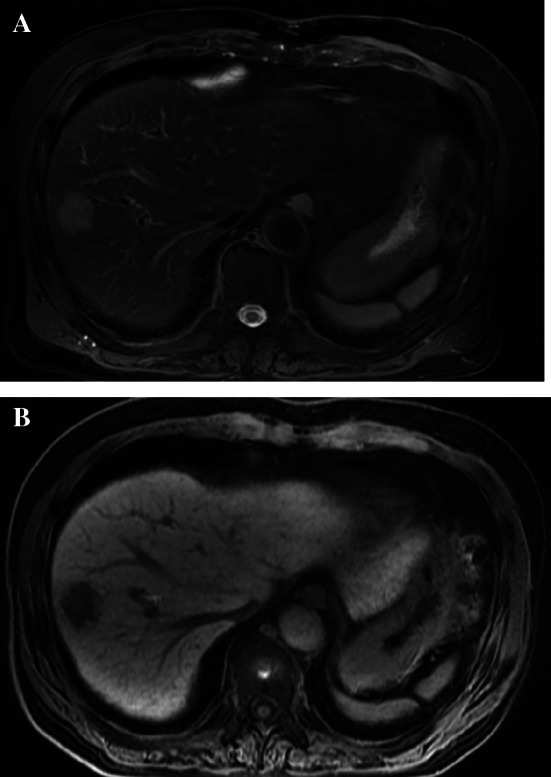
A, B Gadolinium–ethoxybenzyl-diethylenetriamine penta-acetic acid-enhanced magnetic resonance imaging showing a tumor as a hyperintense lesion without central filling on the T2-weighted image (arrow) and a hypointense lesion in the hepatobiliary phase (arrow)
